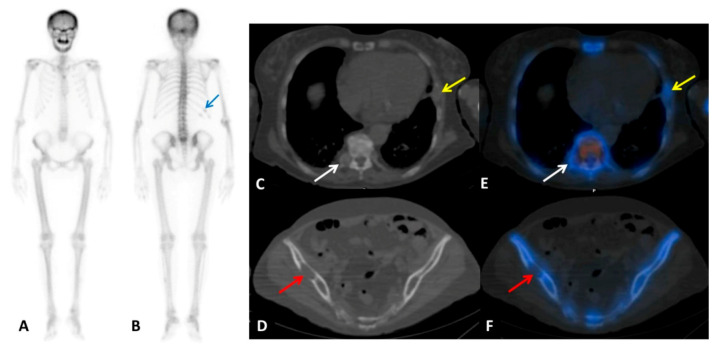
Tc-99m-MDP bone scintigraphy, performed to investigate the unknown bone lesion, showed diffusely increased radiotracer uptake throughout the axial and appendicular skeleton (anterior (A) and posterior (B) planar views). Especially intense activity is noted in the mandible and maxilla with absent renal and bladder activity, suggesting a “metabolic super-scan” [4]. Of note, the mild uptake in the posterior arch of 11th–12th right ribs (blue arrow) is to be referred to a recent trauma reported in the medical patient history. Additional SPECT/CT acquisition (C–F) demonstrated no osteo-tropic radiopharmaceutical uptake in the right D5 costovertebral joint (white arrows) and in the left 6th rib (yellow arrows), as well as in the right iliac wing (red arrows) lytic lesions detectable on co-registration CT images.
